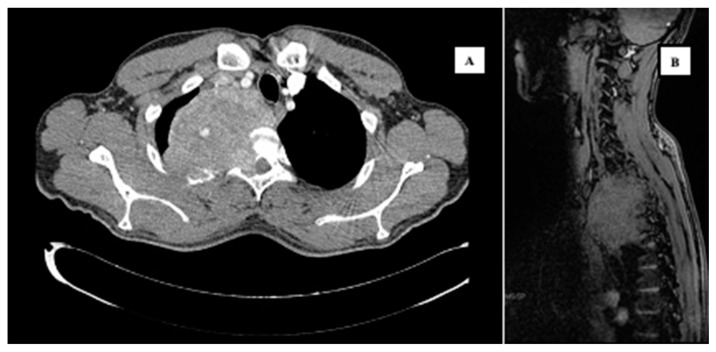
Images of the mass (July 2022) by CT with contrast (A) and by MRI (B), showing infiltration in the ipsilateral posterior third costal arch and in the right T2 and T3 vertebral hemibody, as well as occupation of the conjunctival foramina and right lateral epidural grase in T2–T3 and T3–T4, without invading thecal sac or medullary cord (B).
